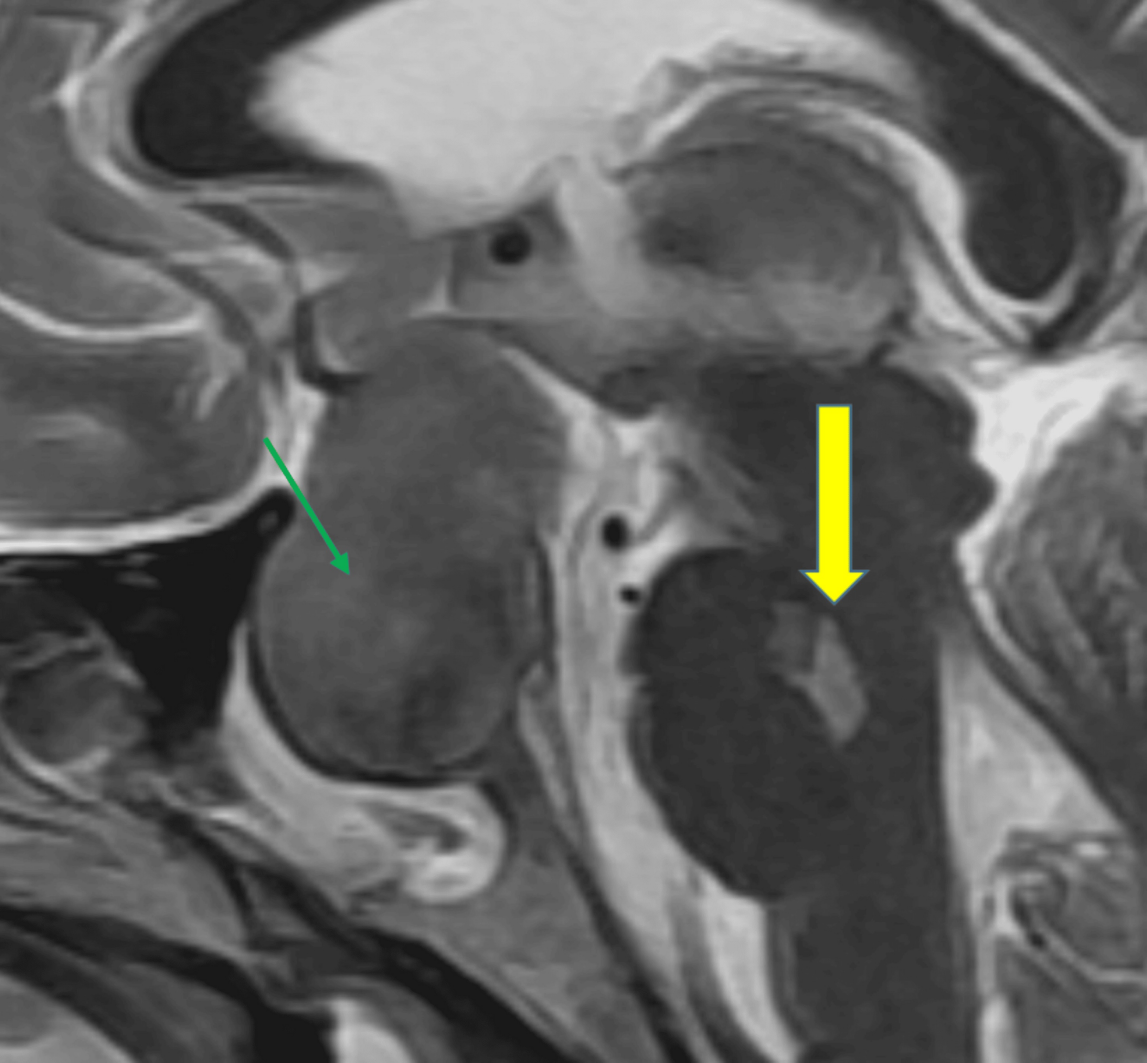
T2 Sagittal MRI Sequence Showing Pontine Myelinolysis (Yellow Arrow) and a Large Pituitary Macroadenoma (Green Arrow) MRI: magnetic resonance imaging
